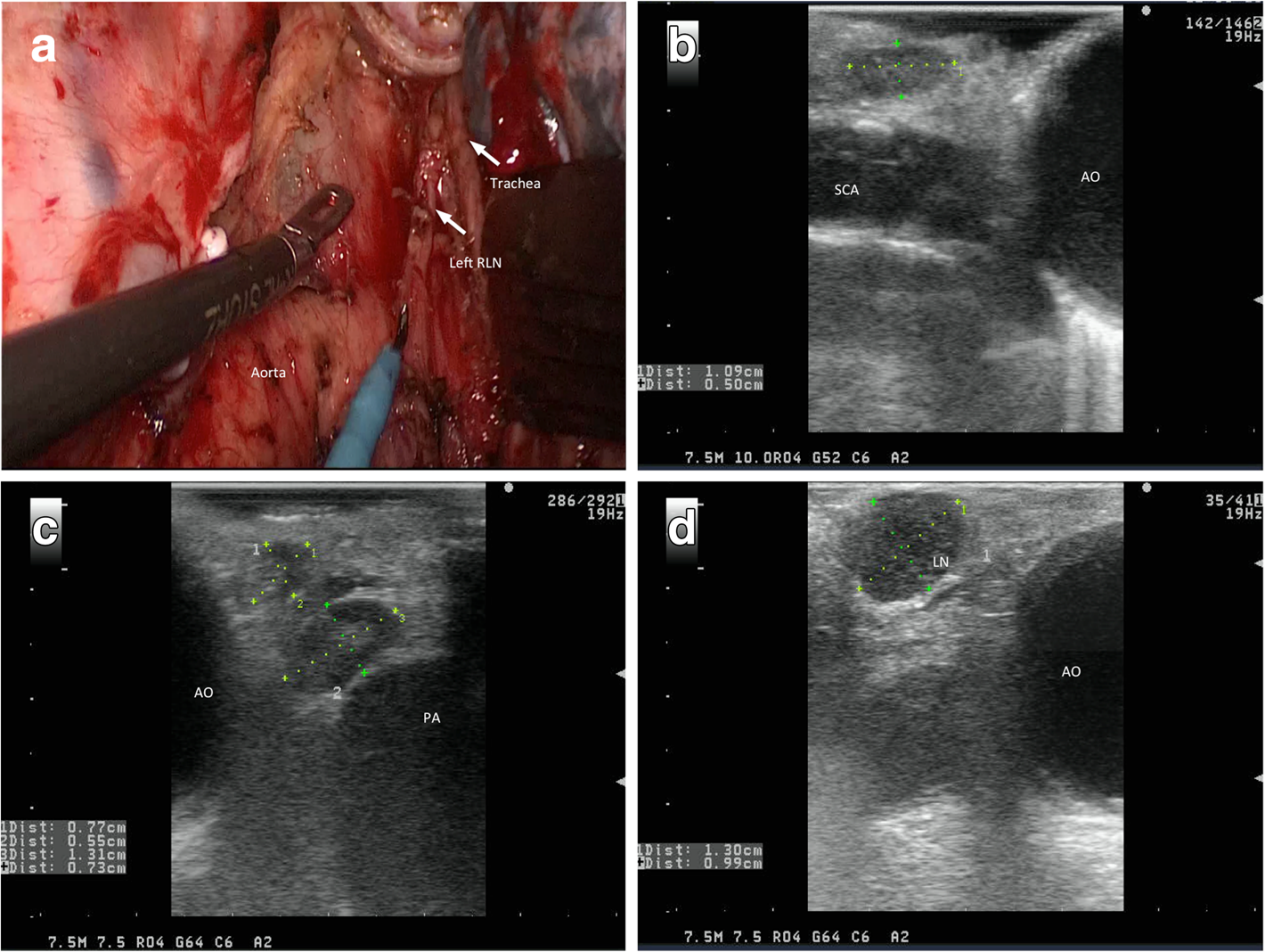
a Completed left thoracic recurrent laryngeal nerve (RLN) node dissection. b Intraoperative ultrasound image showed one non-metastatic lymph node 0.50 cm in diameter located in the left RLN region. c Intraoperative ultrasound image showed the non-metastatic lymph node located in the left RLN region. d Intraoperative ultrasound image showed the metastatic lymph node located in the left RLN region. LN, lymph node; AO, aorta; PA, pulmonary artery; SCA, subclavicular artery
